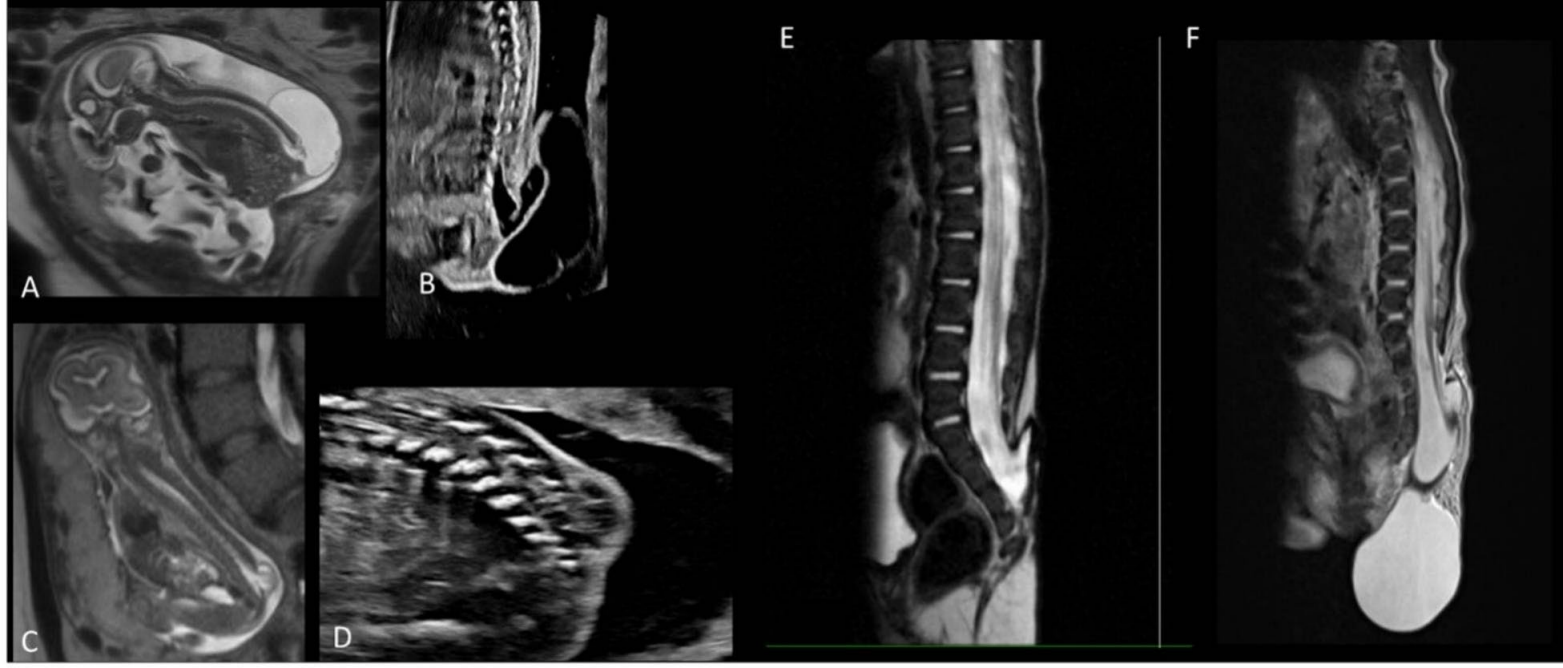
Imaging of sac and no sac defects. A Prenatal MRI of 21-week fetus with myelocystocele (sac) defect. B Prenatal ultrasound of the sagittal spine of the same fetus from image A. C Prenatal MRI of 21-week fetus with lipomyelomeningocele (no sac) defect. D Prenatal ultrasound of the sagittal spine of the same fetus from image C. E Postnatal MRI of 3-month-old with meningocele (no sac) defect. F Postnatal MRI of 1-day-old with meningocele (sac) defect
